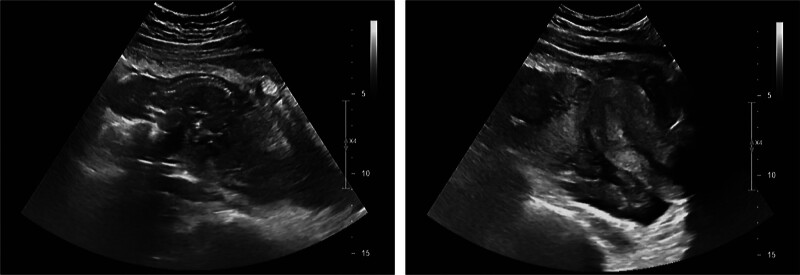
Transabdominal ultrasound of abdominal cavity showing the living fetus with a gestational age of 15 weeks and 3 days.
